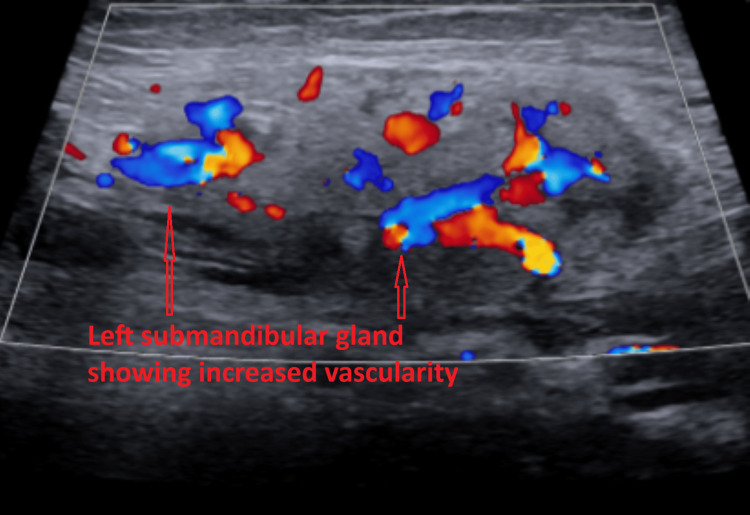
Color Doppler ultrasound image of the transverse view of the left submandibular gland showing increased vascularity
